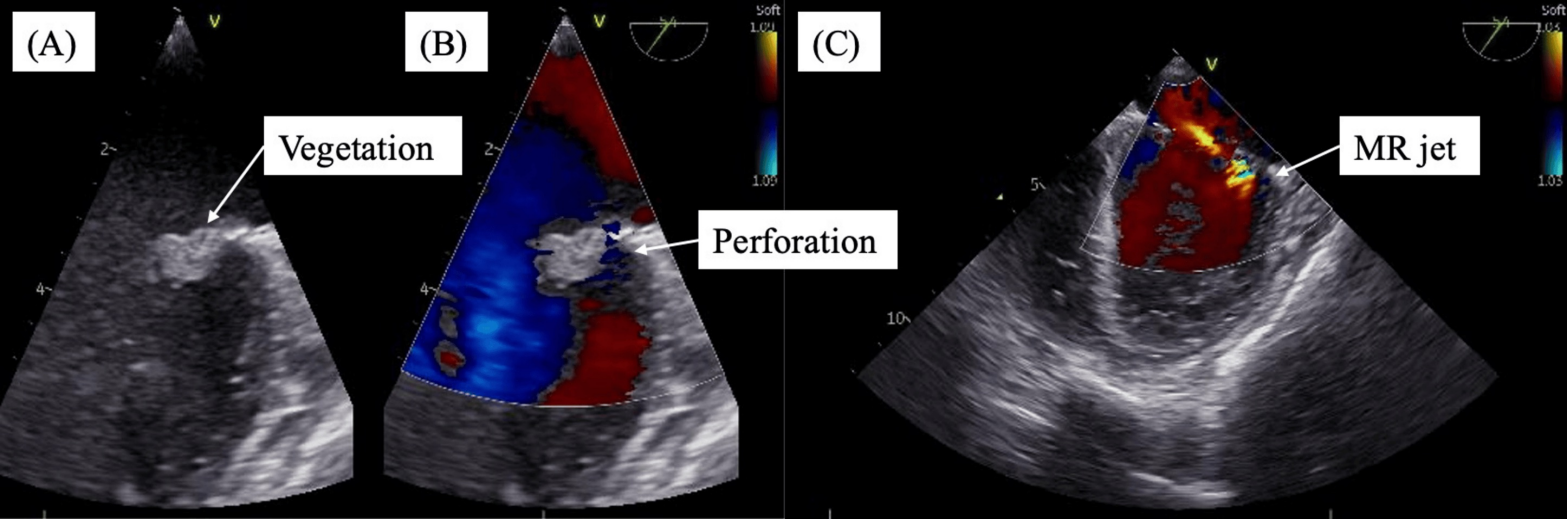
Transesophageal echocardiography on day one showing infective endocarditis (A) B-mode image showing vegetation on the anterior mitral leaflet. (B) Color Doppler image obtained in the same imaging plane demonstrating a mitral regurgitation jet through an anterior mitral leaflet perforation. (C) Additional Color Doppler view demonstrating mitral regurgitation.
